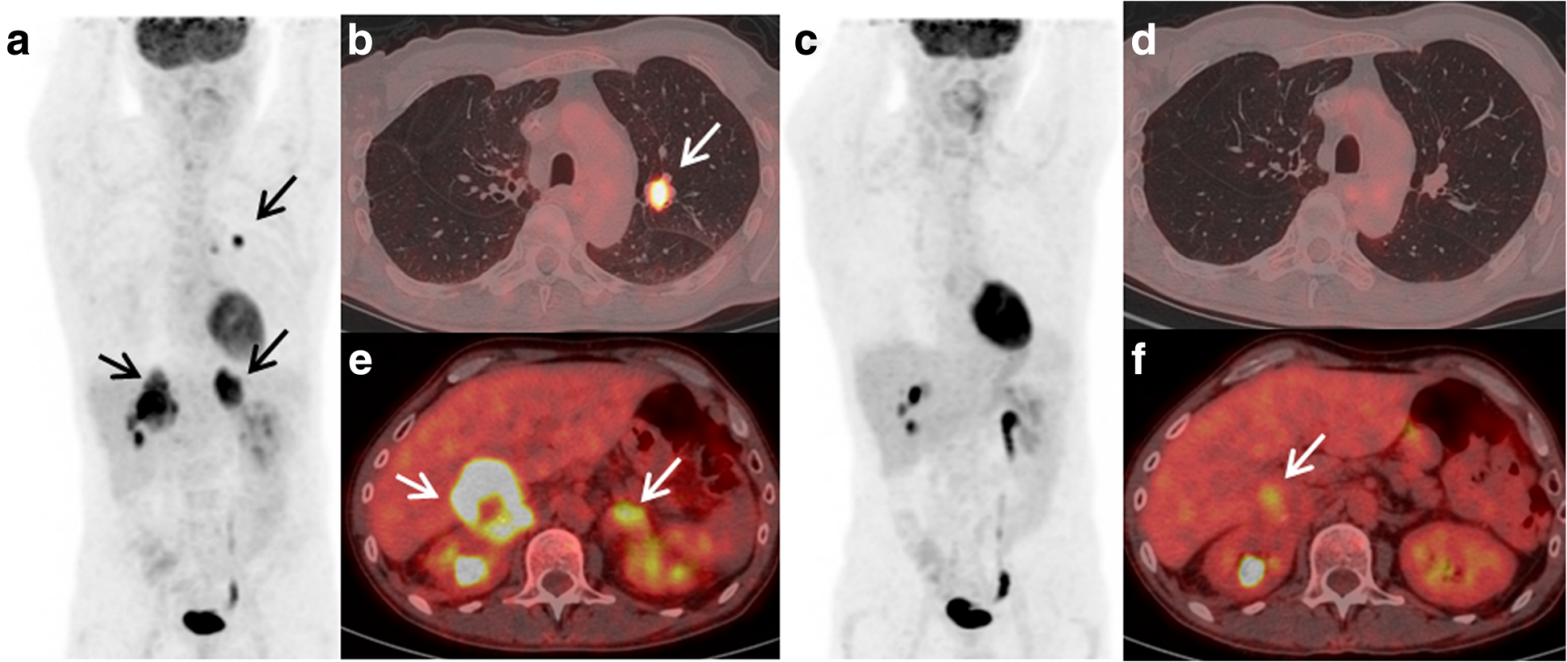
A 64-year-old patient with metastatic lung adenocarcinoma was treated with a second line of nivolumab. SCAN-1 shows lung nodules with adrenal metastases (a, maximum intensity projection (MIP) image, arrows; b, e axial fusion). SCAN-2 at 8 weeks of treatment (4 cycles) showing partial metabolic response (PMR): complete metabolic response on the lungs and left adrenal lesions (c MIP, d axial fusion) but persistent and significant FDG uptake on the right adrenal lesion (f arrow). The classification was PMR according to iPERCIST. Nivolumab treatment was maintained for 10 months, and at the last follow-up, the patient was still alive (survival of 23.8 months)
